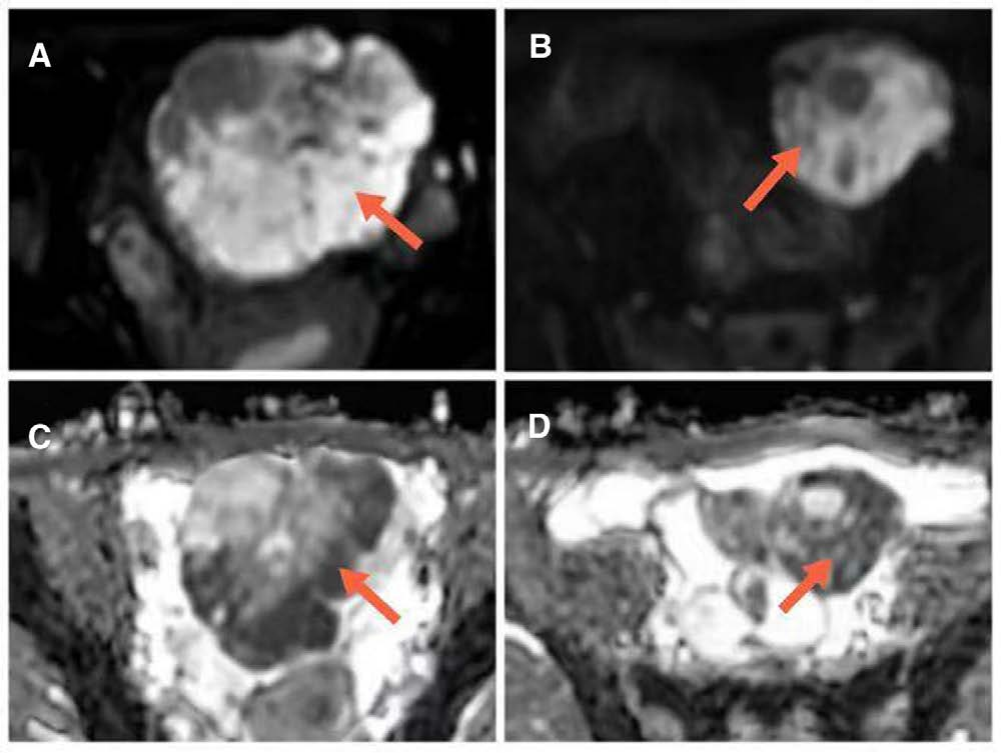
(A and B) When *b* = 800s/mm^2^, the solid component showed a slightly hyperintense signal on DWI images. (C and D) ADC images showed a hypointense signal.
